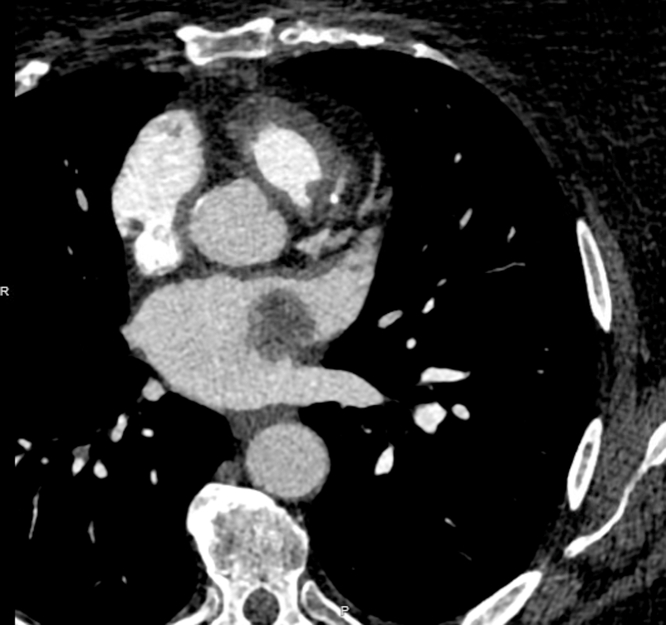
Chest Computed Tomography Showing Filling Defect Measuring Up to 2.2 × 2.1 cm in the Right Atrium Which Appears Pedunculated From the Lateral Atrial Wall
